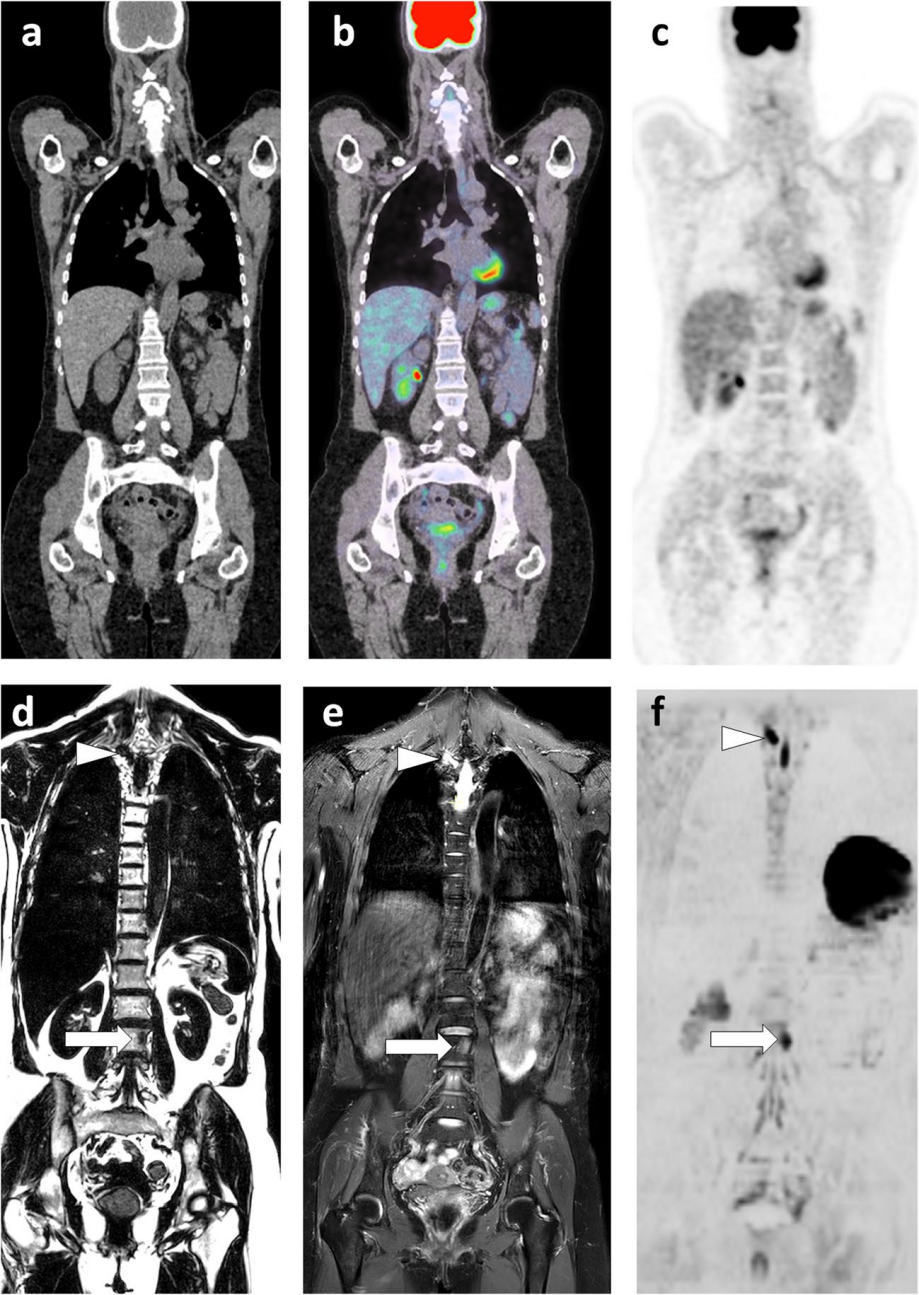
63-year-old woman with lobular carcinoma of the breast and increased tumor marker: comparison of WB-MRI and FDG-PET/CT. a–c Coronal PET/CT images (CT, fusion, PET): absence of evident lesion. d–f Corresponding coronal WB-MRI sections: fat-only (FO) (d) and water-only (WO) (f) images of a T2-weighted Dixon sequence, and coronal high *b*-value (inverted grayscale window, *b* = 1000 s/mm^2^) DWI image (f): bone marrow foci (metastases) at the left corporeo-pedicular junction of L3 (arrow) and the right transverse process of T3 (arrowhead). In total, three metastases were identified by this examination
